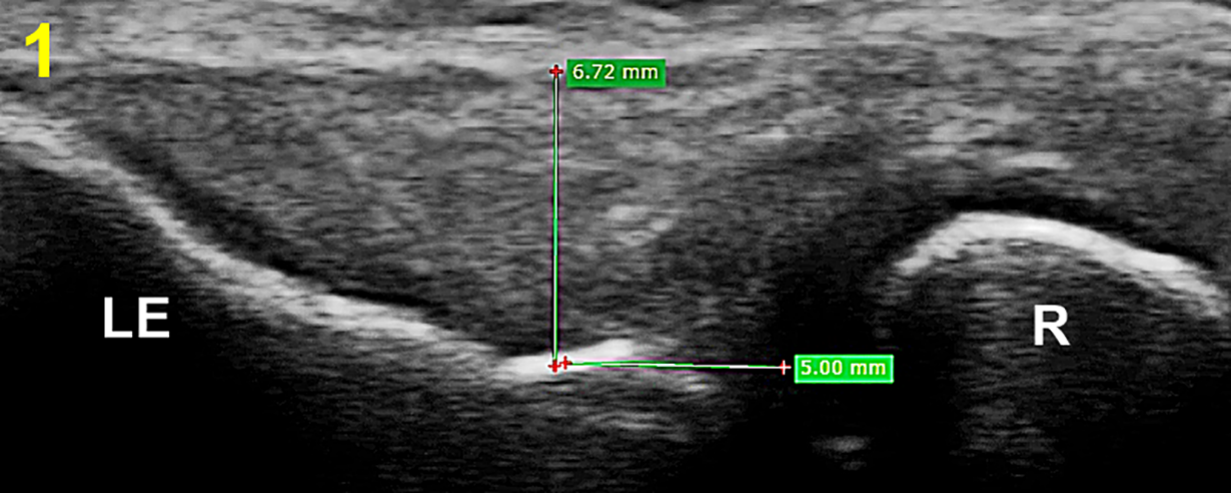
Longitudinal ultrasound view demonstrating thickness measurement (mm) of the common extensor tendon. LE: lateral epicondyle, R: radius.
